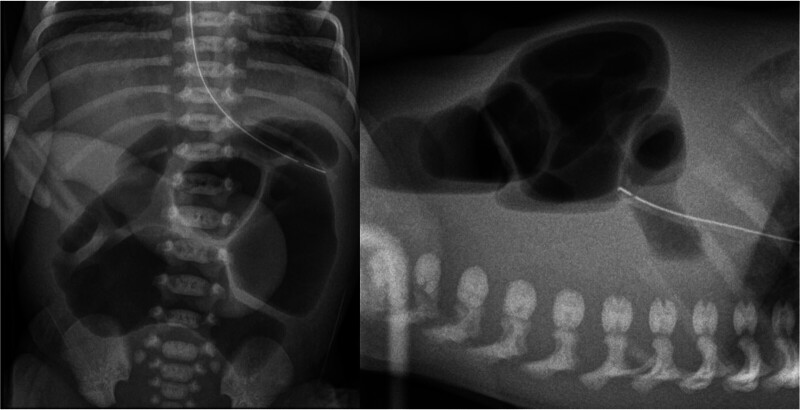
Abdominal X-ray with dilated bowel and air–fluid levels.
